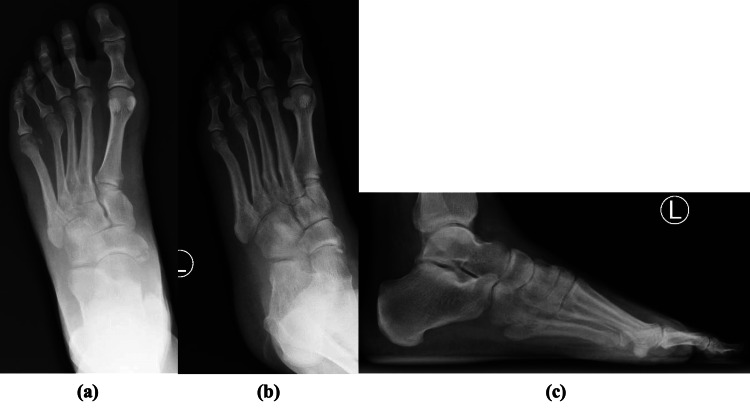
Weight-bearing anteroposterior (a), oblique (b), and lateral (c) radiographs at the 10-week follow-up appointment.
